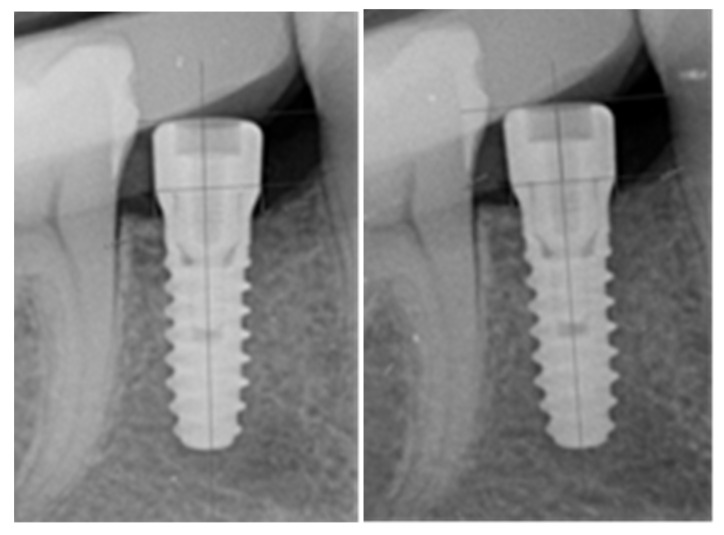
Periapical X-rays at weeks 0 (T0) and 8 (T8), performed with a silicone key and parallelization technique.
